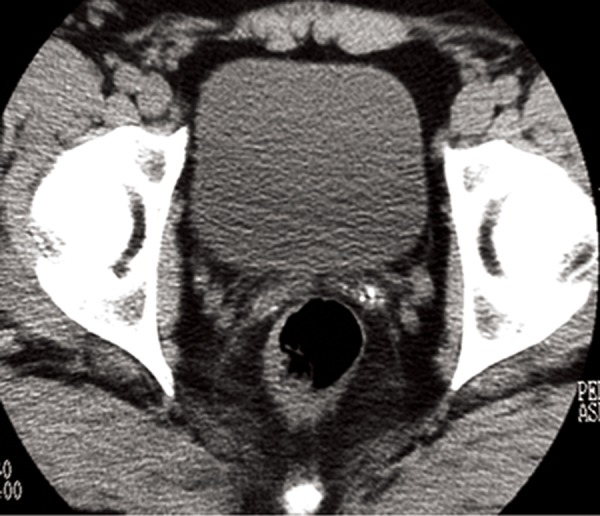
Pelvic HRCT: microliths in the seminal vesicles. HRCT; High resolution computerized tomography scan.
